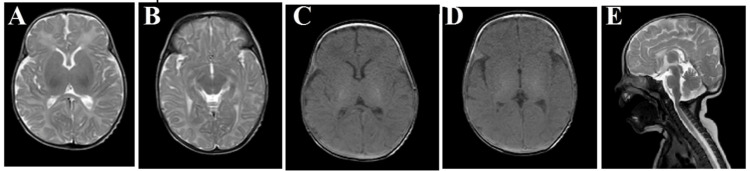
Brain MRI (A,B) (T2-weighted) showing a coronal view of the brain. (C,D) (T1-weighted) showing a coronal view of the brain. E (T2-weighted) showing a sagittal view of the brain.
